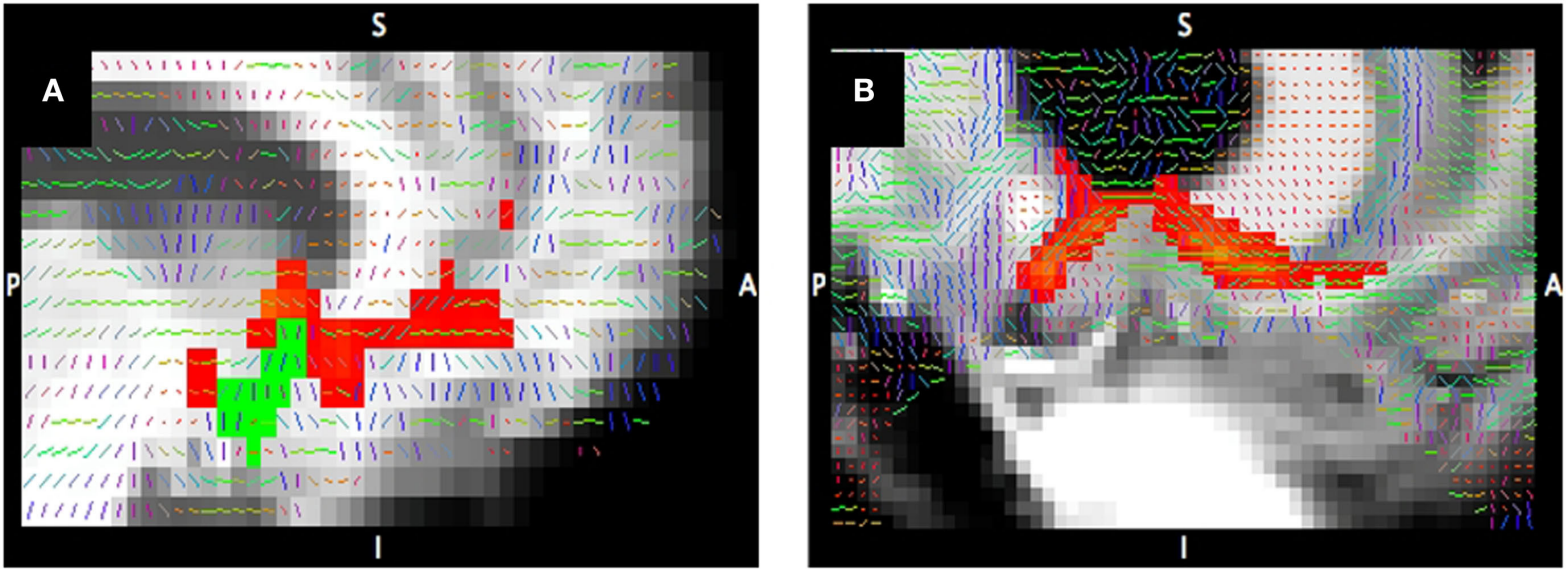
Tractography at 3-T compared to 7-T. Tracts connecting the nucleus accumbens (shown in green) to the ventral prefrontal cortex from diffusion-weighted imaging at 3-T (A) and 7-T (B). Tract probability map >1% of streamlines (red) corrected for distance; primary diffusion direction overlaid on T1-weighted image.
